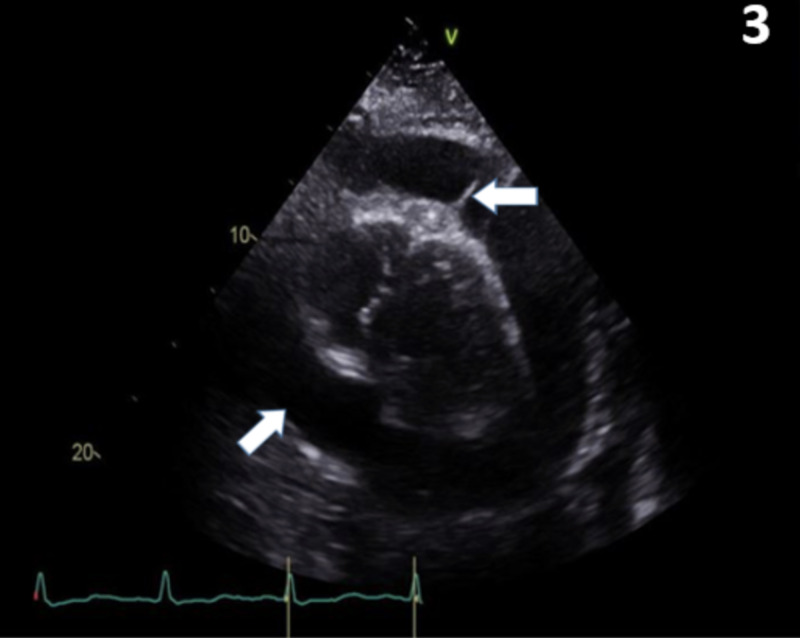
Transthoracic echocardiography shows a large (2 cm) pericardial effusion circumferentially surrounding the heart with fibrinous focal strands (white arrows).
